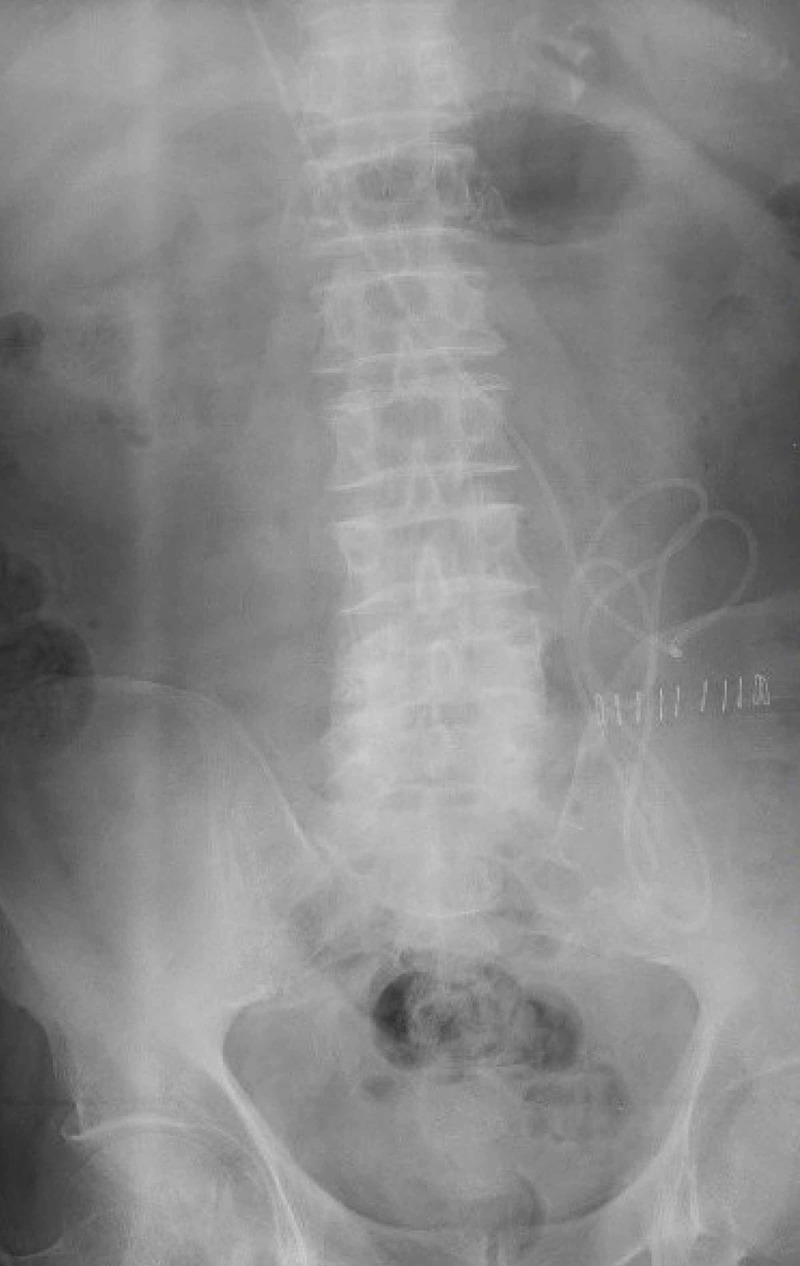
Post-operative abdominal X-ray confirms the location of distal catheter in the peritoneum.
